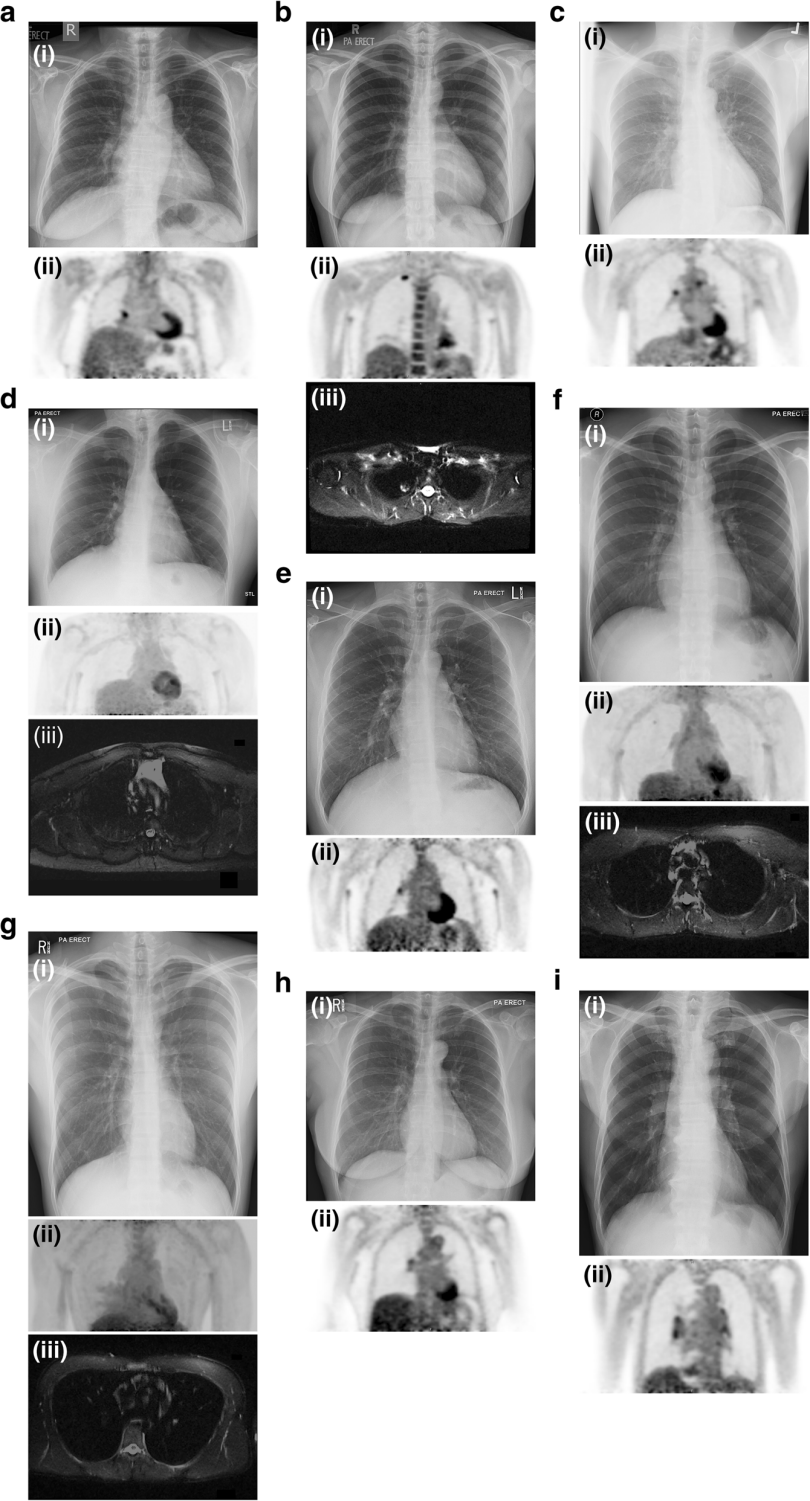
Abnormal imaging findings in TB contacts. a. Participant 2. 61y female, asymptomatic, (i) CXR: normal (ii) PET/MRI: FDG-avid right mediastinal lymph node (SUVmax 3.2); b. Participant 3. 35y female, asymptomatic, (i) CXR: normal (ii) PET/MRI: Spherical FDG-avid lesion in the apex of the right lung (SUVmax 3.9) (iii) Increased signal observed on MRI (HASTE); c. Participant 8. 54y male, asymptomatic, (i) CXR: normal (ii) PET/MRI: FDG-avid right hilar lymph node (SUVmax 2.4) and mediastinal lymph node (SUVmax 2.5); d. Participant 9. [914] 46y male, asymptomatic, (i) CXR: normal (ii) PET/MRI: Diffuse left upper lobe parenchymal uptake (SUVmax 2.2) (iii) No underlying MRI changes; e. Participant 12. 30y male, 1 week of mild non-productive cough, (i) CXR: normal (ii) PET/MRI: Right hilar lymph node (SUVmax 2.6); f. Participant 14. [922] 25y male, asymptomatic, (i) CXR: normal (ii) PET/MRI: RUL parenchymal uptake (1.3) (iii) Small underlying lesion identified on MRI images; g. Participant 16. [924] 22y male, 1 week of mild non-productive cough, (i) CXR: normal (ii) PET/MRI: Patchy right middle lobe parenchymal uptake (2.8), (iii) Bilateral underlying patchy changes identified on MRI. The area of right middle lobe uptake overlies an area of MRI abnormality; h. Participant 18. 46y female, asymptomatic, (i) CXR: normal (ii) PET/MRI: Right hilar lymph node (SUVmax 2.2); i. Participant 30. 66y male, asymptomatic, (i) CXR: normal, (ii) PET/MRI: several FDG-avid hilar lymph nodes (SUVmax = 2.8). (Note that all PET images are SUV images scaled between 0 (white) and 3 (black))
